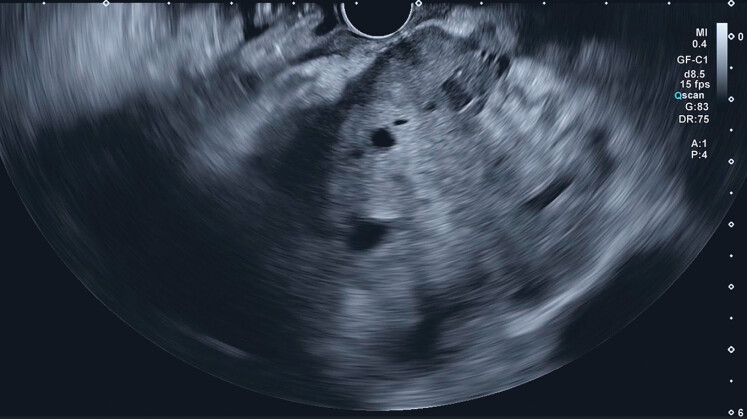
Endoscopic ultrasound B-mode image of the pancreatic body mass (Aplio i800; Canon Medical Systems, Tochigi, Japan), showing a hypoechoic and heterogeneous mass in the pancreatic body, with suspected necrotic areas.
